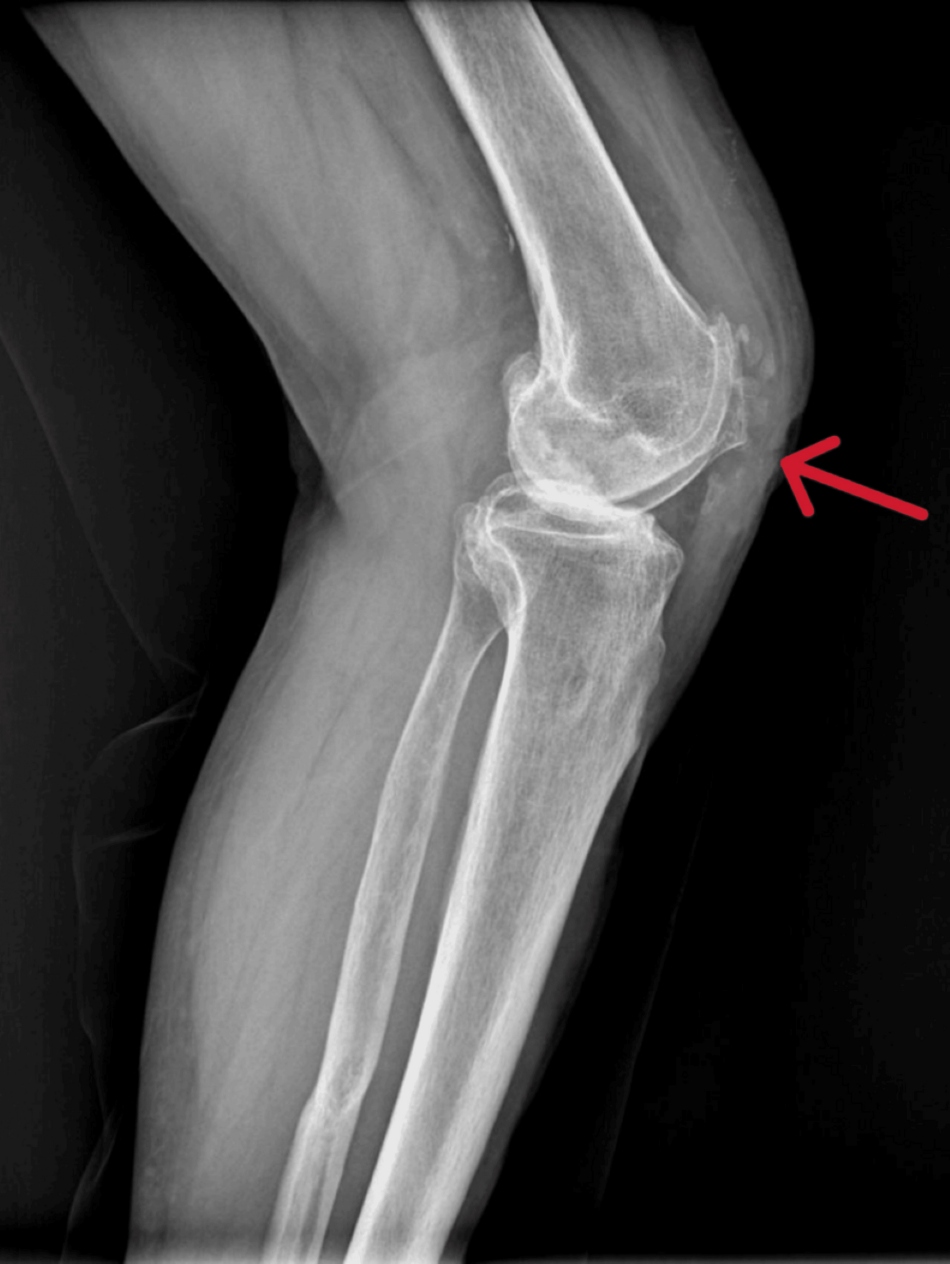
Preoperative Lateral X-ray of the Left Knee (Weight Bearing, Arrow)
